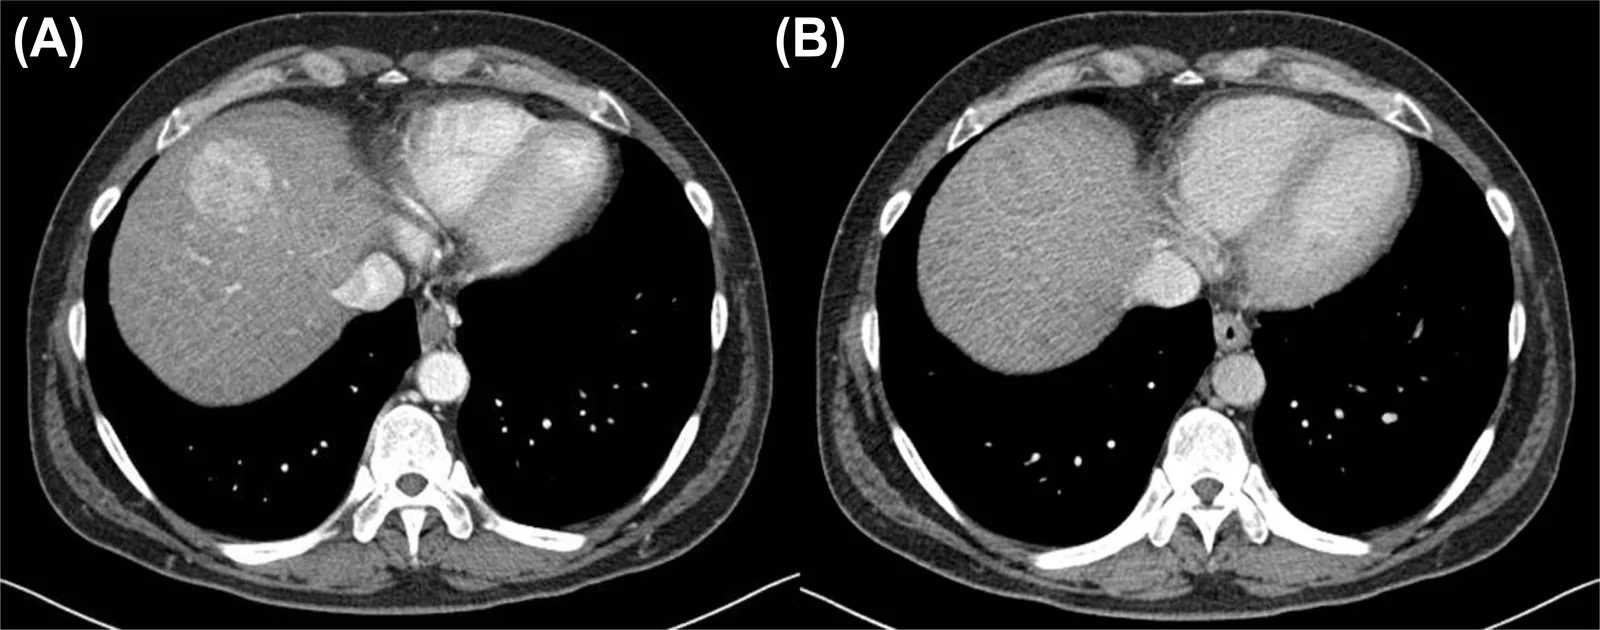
(A) The arterial phase of contrast-enhanced CT shows marked hyperenhancement of the hepatic lesion. (B) The delayed phase image demonstrates a washout appearance of the lesion.
